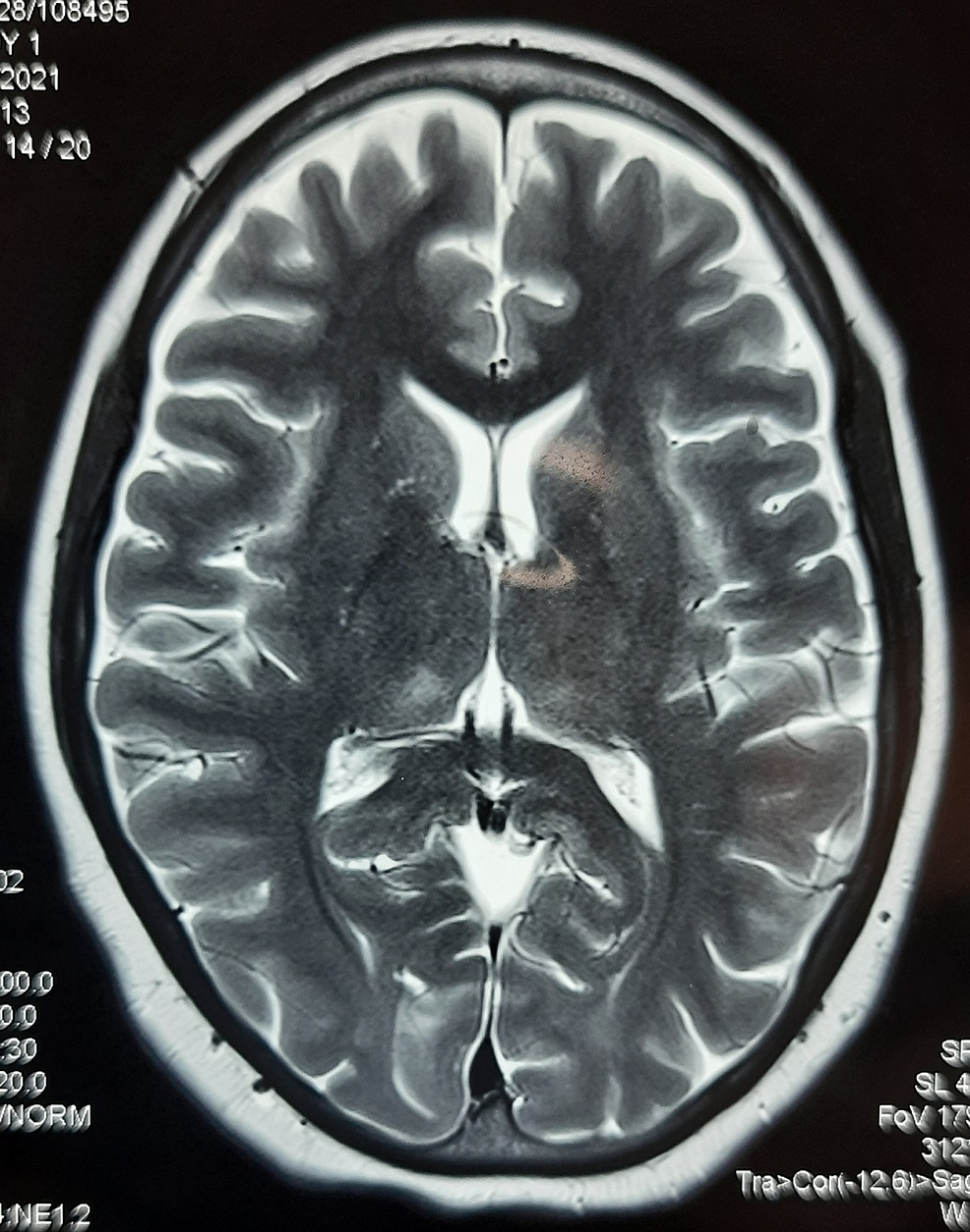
T2 MRI showing subtle bilateral thalamus involvement (right>left)
